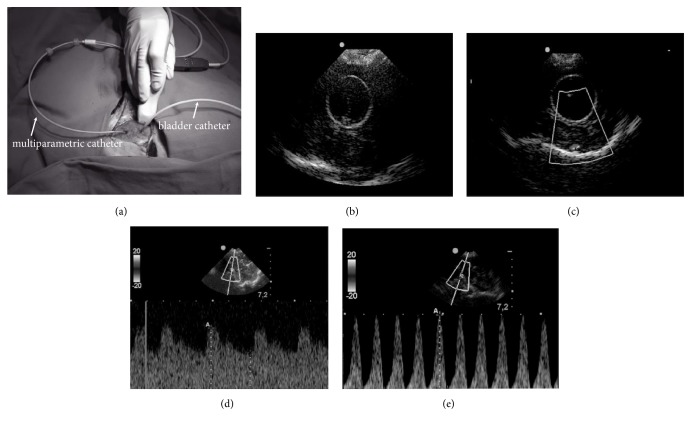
(a) Ultrasound transducer under the lateral hole, a multiparametric catheter in the anterior hole, and a bladder catheter in the posterior hole. (b and c) B-mode ultrasound duplex demonstrating the inflated balloon. Doppler mode was used to obtain CBFV before (d) and after balloon inflation (e).
